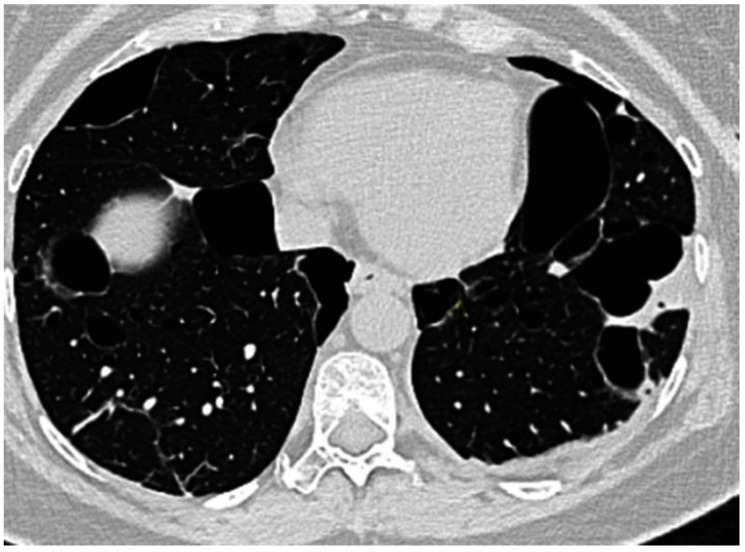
Chest CT, Case 7: bilateral, basally predominant, medial/subpleural thin-walled cysts with paramediastinal involvement.
